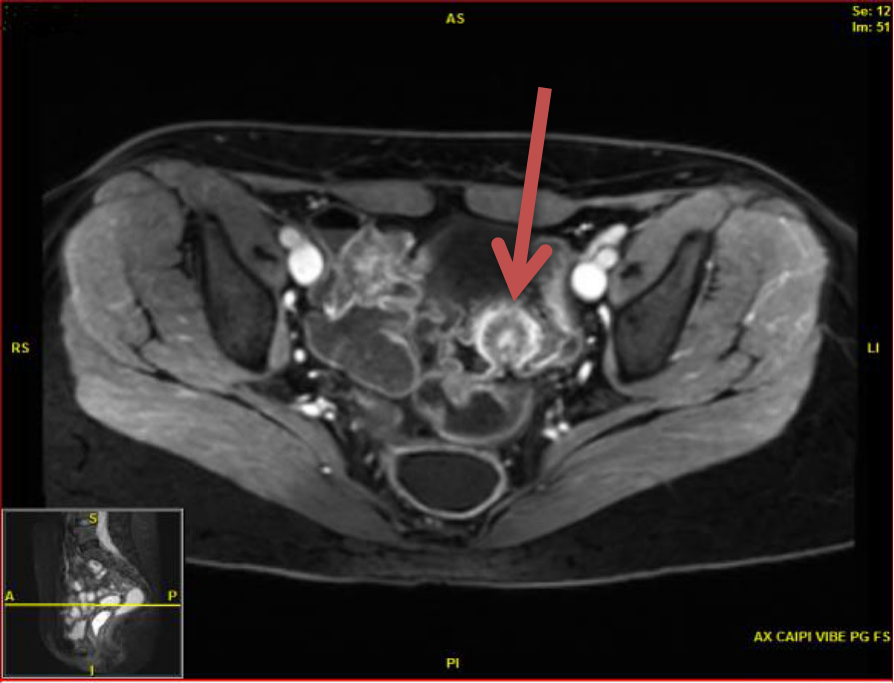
Transverse T2-weighted magnetic resonance imaging section of pelvis with recto-sigmoid colon endometriosis (*red arrow*)
